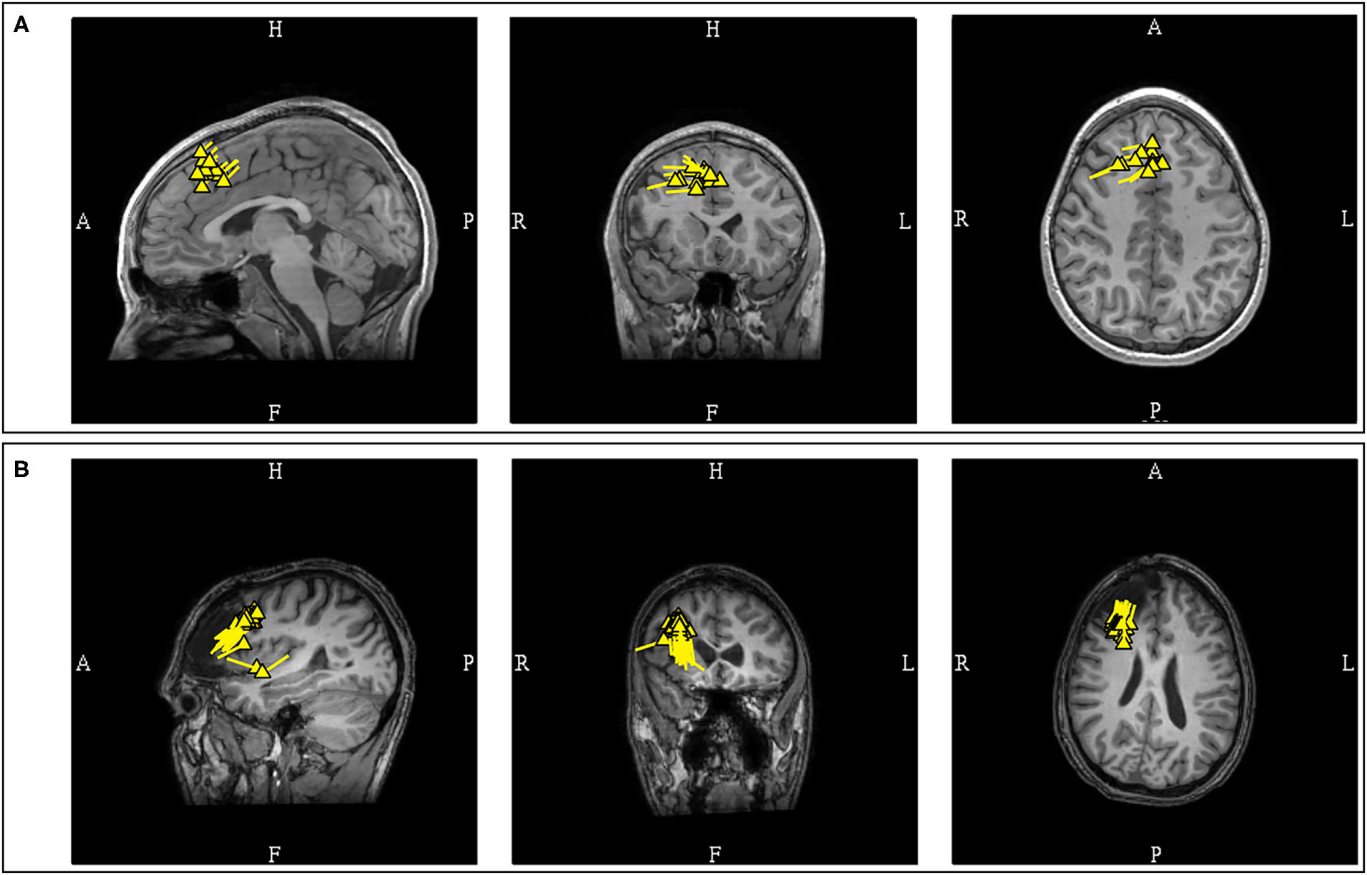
(A) MEG-MRI coregistration summary demonstrating predominantly interhemispheric frontal dipoles. The uniform vector orientation toward the right suggests a right hemispheric source lateralization. Intracranial recording confirmed the finding, with seizure freedom for 5-years after right frontal resection. (B) This patient developed late seizure recurrence, with tight and uniform dipole cluster at the resection margin. Note the dipole orientation suggestive of positivity at the resection surface as a result of disruption of normal cortical laminar organization.
